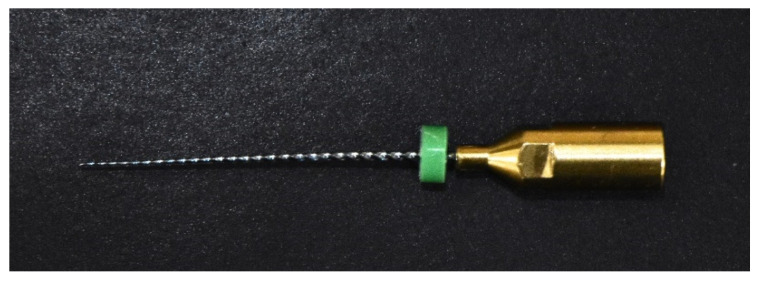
Ni-Ti ultrasonic instrument.
